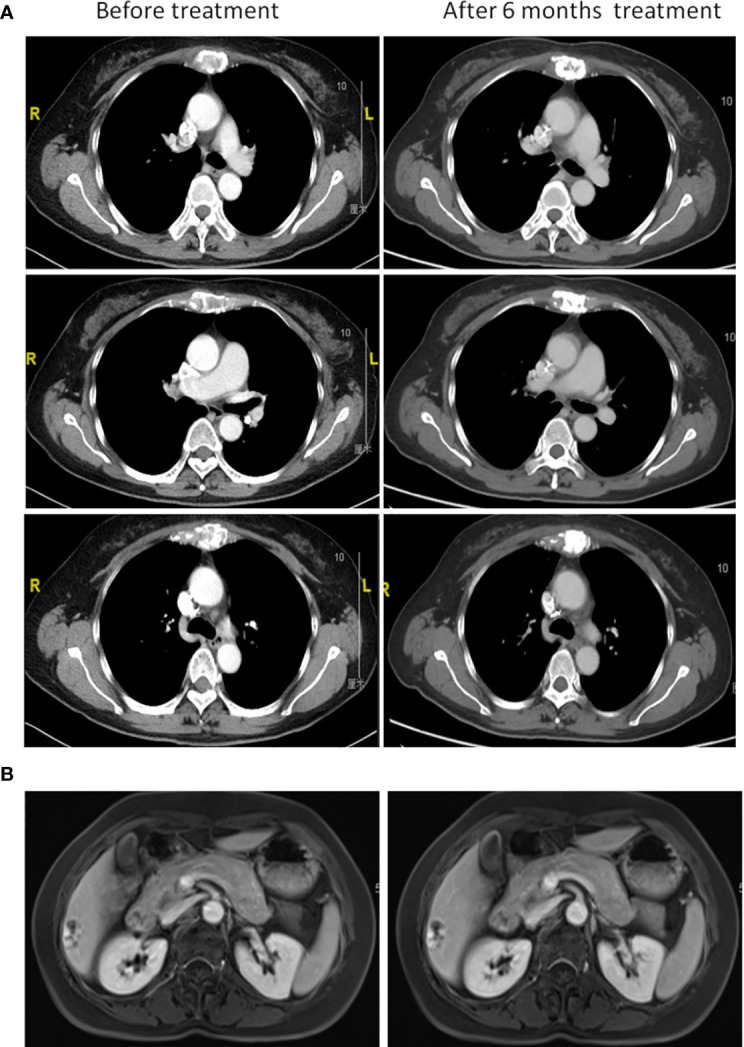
Clinical course after initiation of standard chemotherapy. (A) Chest computed tomography and (B) liver magnetic resonance imaging before chemotherapy treatment and after 6 months.
